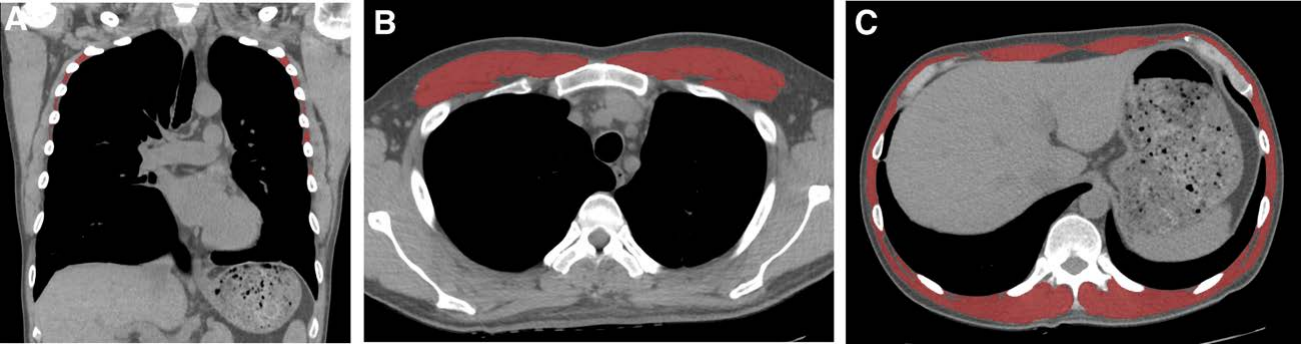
Muscle area measurement using non-contrast chest CT in patients with COVID-19. The region of interest (ROI) was manually delineated along the outermost border of the muscle, and muscle areas from −29 to 100 HU were displayed in pink and subsequently quantified using CT histogram analysis. (A) On a coronal image, the cross-sectional areas of both 3^rd^ to 8^th^ intercostal muscles were measured at the midline level. (B) On an axial image, the cross-sectional areas of both pectoralis major and minor muscles were measured at the 4^th^ level of the thoracic vertebra. (C) On an axial image, the cross-sectional areas of all the muscles were measured at the 12^th^ level of the thoracic vertebra. HU = Hounsfield units.
